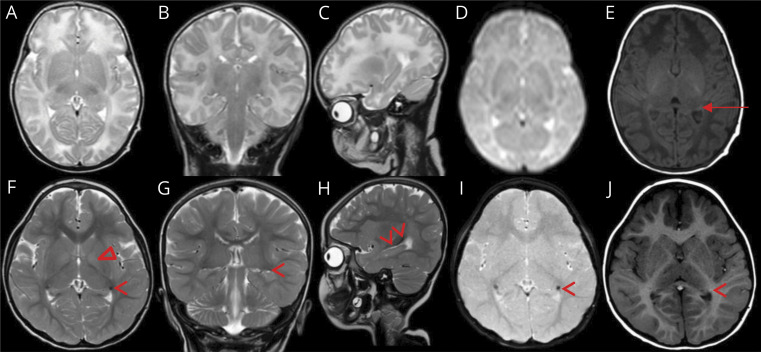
MRI of the index patient (II:3) Images at ages 23 days (A–E) and 4 years (F–J). T2-weighted MRI in axial (A and F), coronal (B and G), and sagittal plane (C and H), axial GRE imaging (D and I), and T1-weighted imaging in axial plane (E and J). At age 4 years, a persistent high signal is perceived in the basal part of the bilateral globus pallidi (Δ) (F), which is not seen at age 23 days (A). The caudate part of the caudate nuclei (arrow head) presents low signal on T2 in all 3 planes (F–H) including GRE imaging (I) with high signal on T1 (J) compared with normal appearance at age 23 days (A–E). Only the normal mature lateral geniculate body of the thalamus (arrow) is seen at age 23 days just medially to the caudate part of the caudate nucleus (E). GRE = gradient echo sequences.
